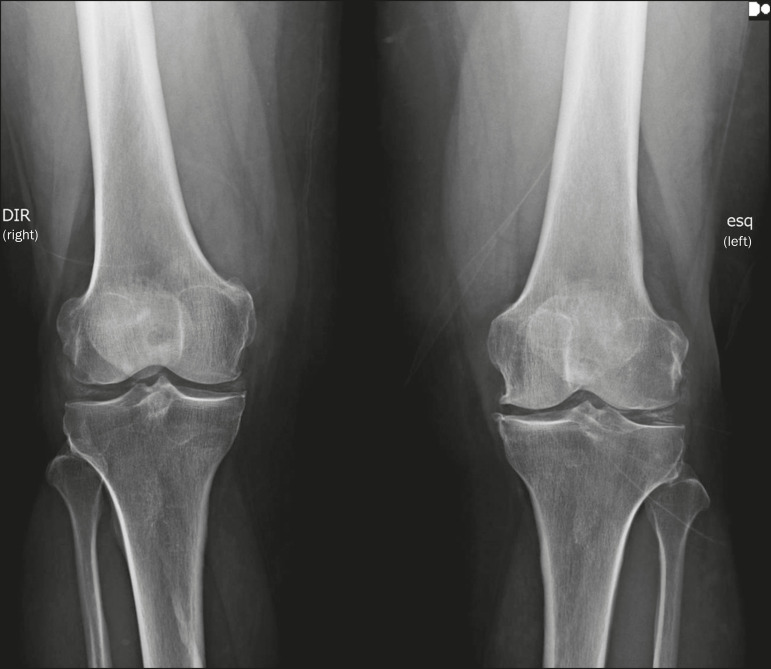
Articular cartilage calcifications in CPPD deposition disease. Frontal X-ray of the knee showing small calcifications in the articular hyaline cartilage and in the meniscus. Note the linear, stratified aspect of the calcifications.
